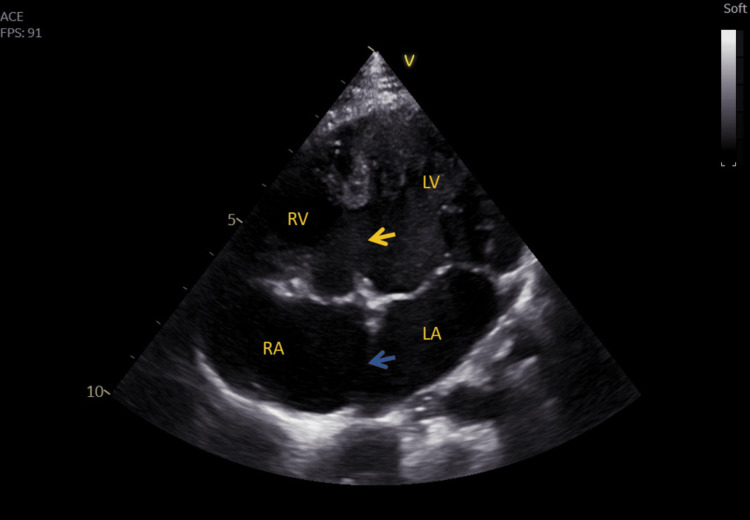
Transthoracic echocardiogram, apical four-chamber view Left ventricle is slightly dilated; right ventricle is small. Yellow arrow: large interventricular septum defect; blue arrow: ostium secundum interatrial septum defect; RA: right atrium; LA: left atrium; RV: right ventricle; LV: left ventricle
